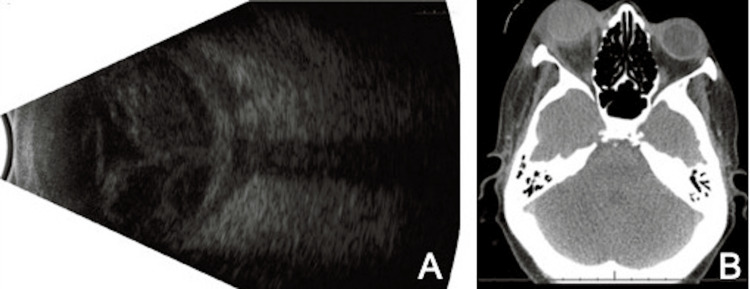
Imaging of Suprachoroidal Hemorrhage A) B-scan ultrasonography of choroidal hemorrhage demonstrating a “T sign” and “kissing choroidals” with associated vitreous hemorrhage. B) Axial CT scan of orbit revealing right peri-global edema and inflammation with diffuse vitreous humor attenuation which in the setting of proptosis, resistance of retropulsion, and new adduction defect raised suspicion for orbital cellulitis. CT: Computed tomography
